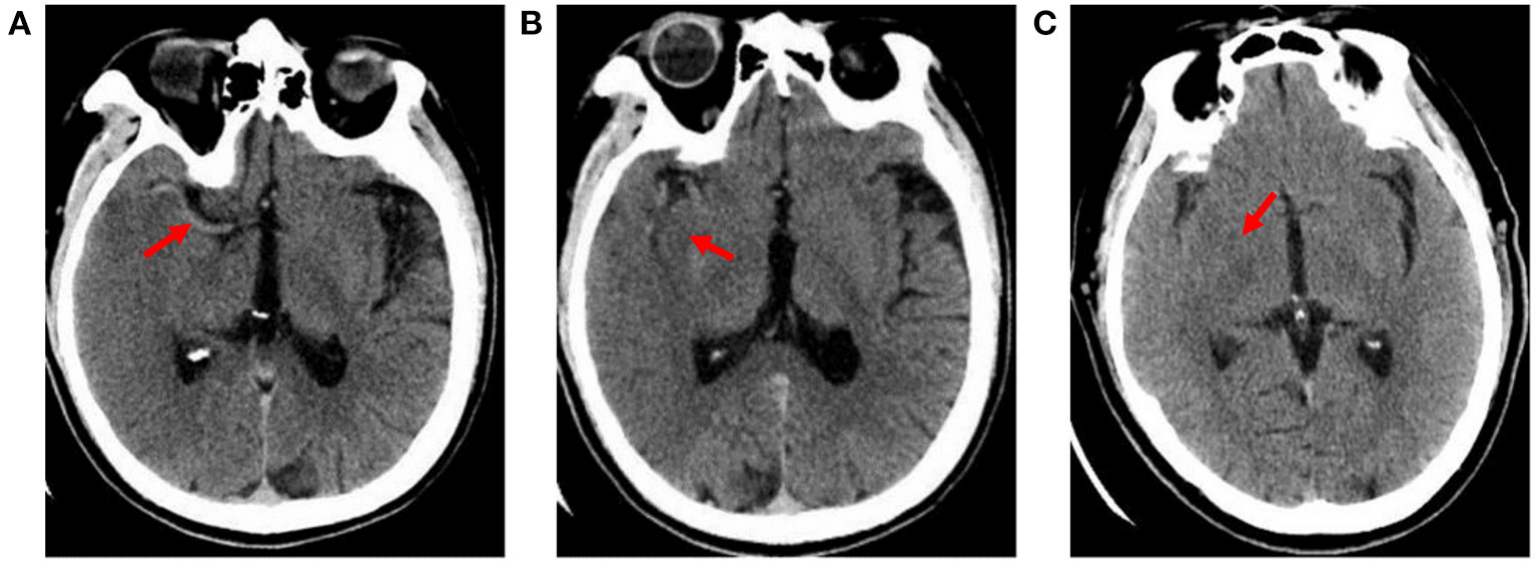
Early imaging signs on CT. (A) hyperdense middle cerebral artery sign: the M1 segment of the right middle cerebral artery shows high density (X-ray uptake value of 64 HU); (B) insular ribbon sign: the gray-white matter interface of the right insular zone disappears, and the cerebral sulcus becomes shallow; (C) obscuration of the lentiform nucleus: the right lentiform nucleus structure is blurred, and the density is reduced (the CT average value is 27.4 HU; the CT average value of the same area on the healthy side is 34 HU).
